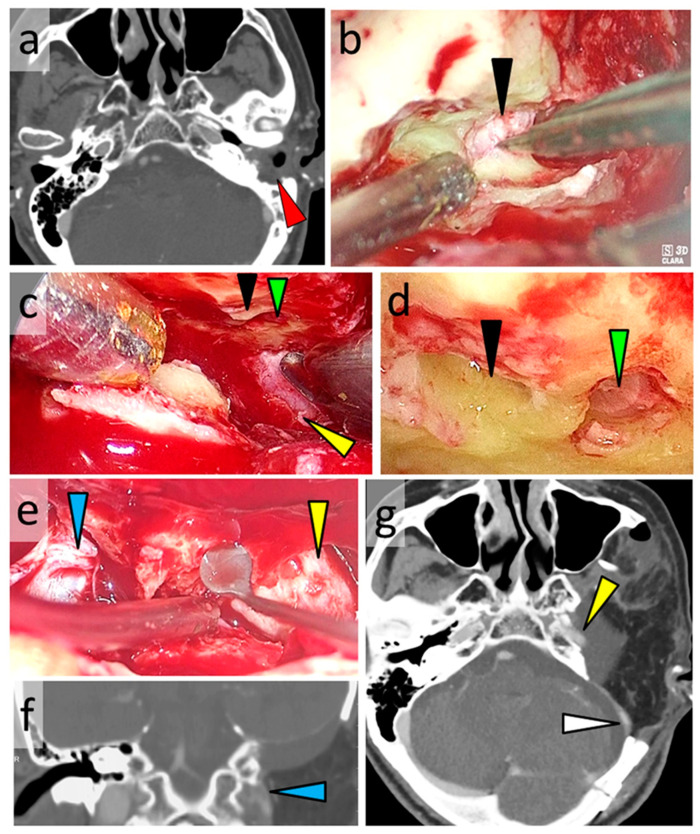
CT images and photographs of Case 2. Subtotal temporal bone resection performed in a patient with external auditory canal squamous cell carcinoma. (a) Preoperative axial CT image showing left external auditory canal carcinoma involving the temporomandibular joint, tympanic cavity, and mastoid air cells. (b) Intraoperative exoscopic photographs showing the opened internal auditory canal (black arrowhead). (c–e) Intraoperative endoscopic photographs. (c) The carotid canal is opened following the transection of the internal auditory canal (black arrowhead) and cochlea (green arrowhead). Yellow arrowhead: the internal carotid artery. (d) The 30° angled endoscopic photograph showing the cut end of the internal auditory canal (black arrowhead) and cochlea (green arrowhead). (e) The lateral wall of the carotid canal (yellow arrowhead) and jugular foramen (blue arrowhead) are carefully dissected. (f) Postoperative coronal CT image. Blue arrowhead: opened jugular foramen. (g) Postoperative axial CT image. Yellow arrowhead: opened carotid canal. White arrowhead: sigmoid sinus. CT, computed tomography.
